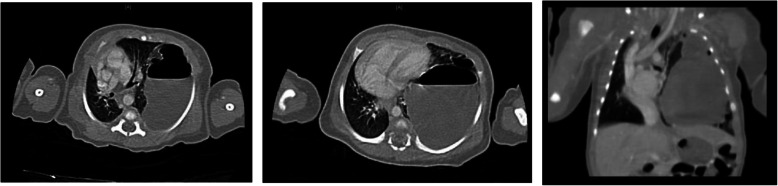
The CT scan showed that the cyst had caused severe compression of the heart, lungs, and mediastinum
